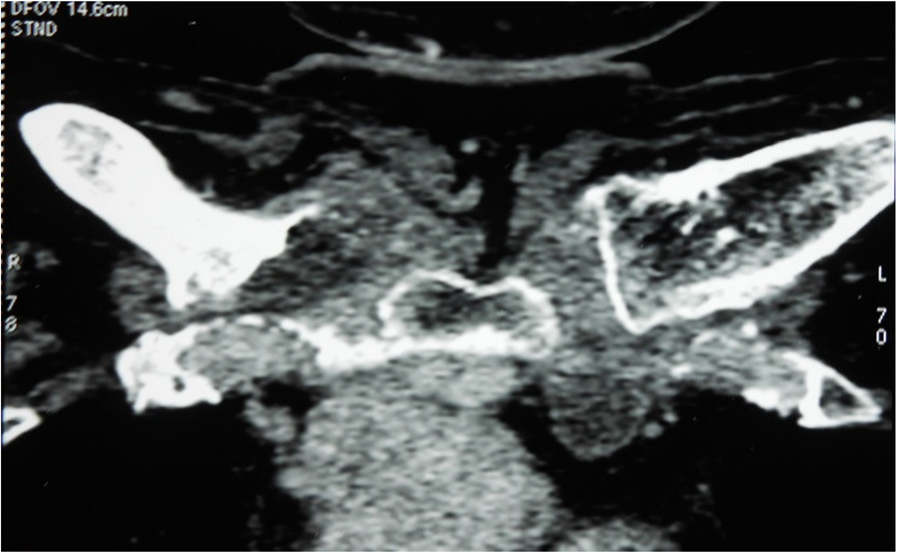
Computed tomography scans of the sternoclavicular joints showing bilateral soft tissue collections
